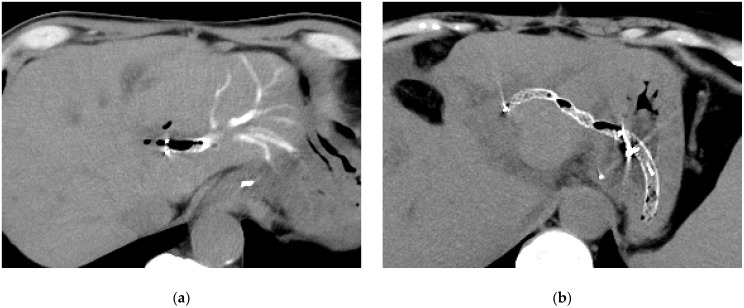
Computed tomography image taken the day after endoscopic ultrasound-guided hepaticogastrostomy (EUS-HGS). (a) The contrast medium remains in the bile duct beyond the HGS stent placement site. (b) There is no residual contrast medium in the bile duct.
